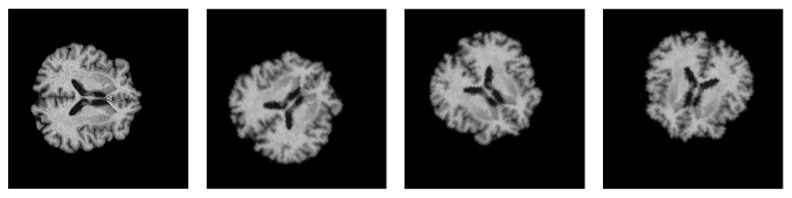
Visualization of the augmented brain MRI images.
